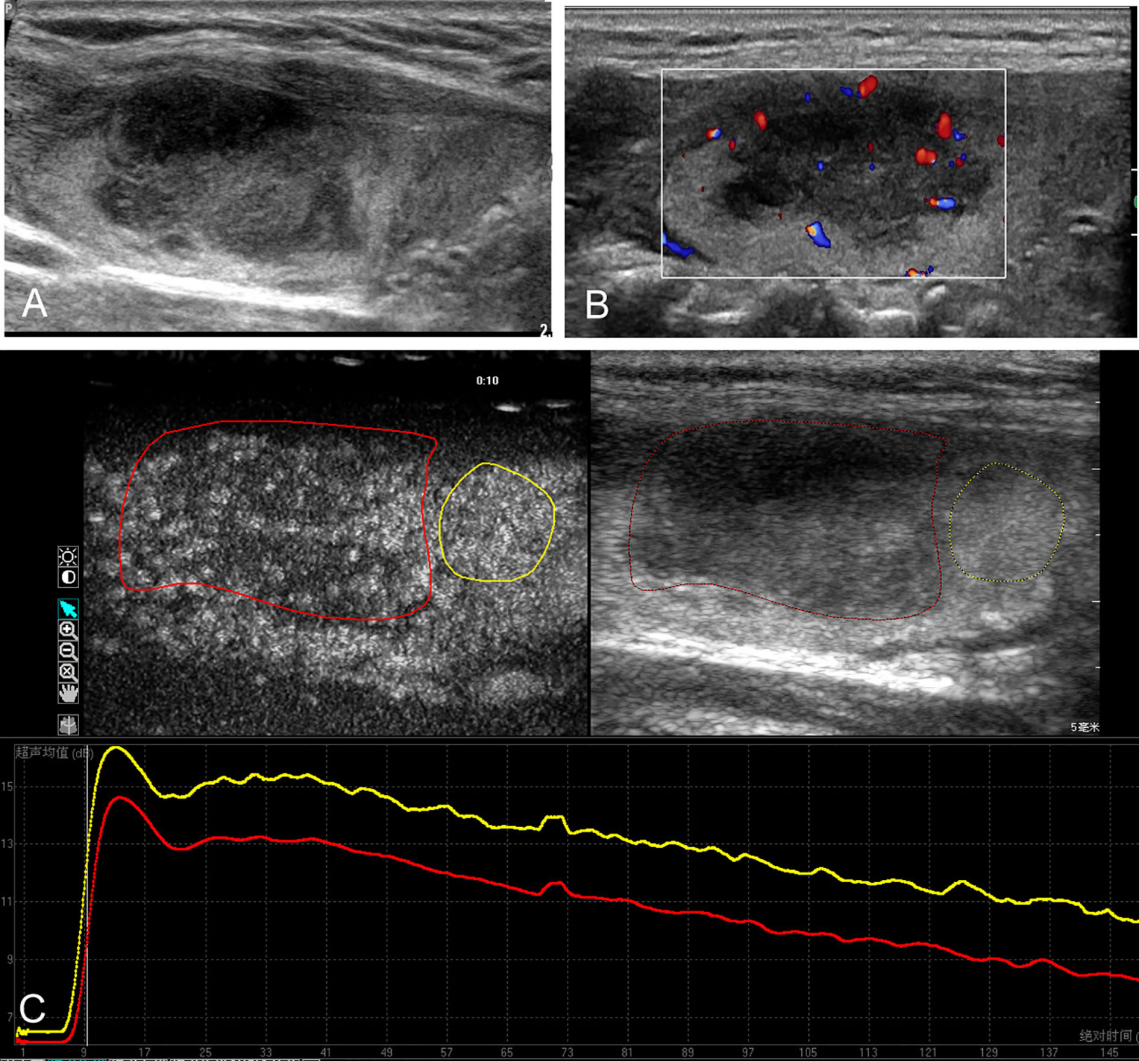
Conventional ultrasound and contrast-enhanced ultrasound images of the primary thyroid lymphoma in the right thyroid lobe of a 67-year-old female with Hashimoto’s thyroiditis background. (A) B-mode ultrasound image of a maximum diameter of 28 mm nodule displayed hypoechogenicity with ill-defined margin. (B) Color Doppler image revealing the internal and peripheral mixed distributed vascularity of the nodule. (C) Contrast-enhanced ultrasound image revealing the heterogeneous hypo-enhancement of the lesion (red circle), with respect to the surrounding parenchyma (yellow circle); time–intensity curves of the lesion (red line) and parenchyma (yellow line) were generated to calculate the corresponding quantitative parameters.
